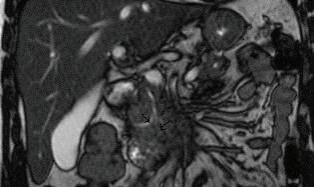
MRI image showing the presence of dorsal pancreatic duct (right) and ventral pancreatic duct (left) that drains into the major papilla
